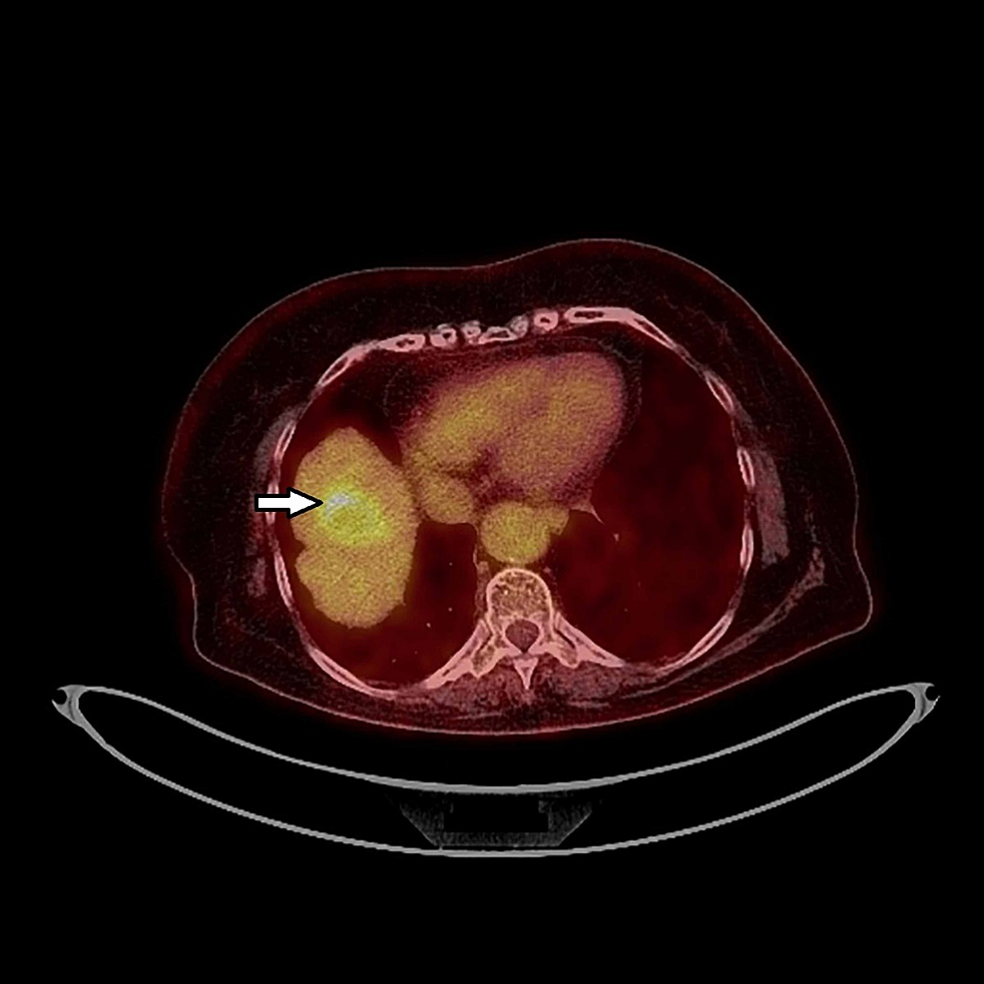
Pre-operative PET-CT showing the single liver metastasis (arrow) from the lung squamous cell carcinoma, in segment VIII. PET-CT: positron emission tomography-computed tomography.
